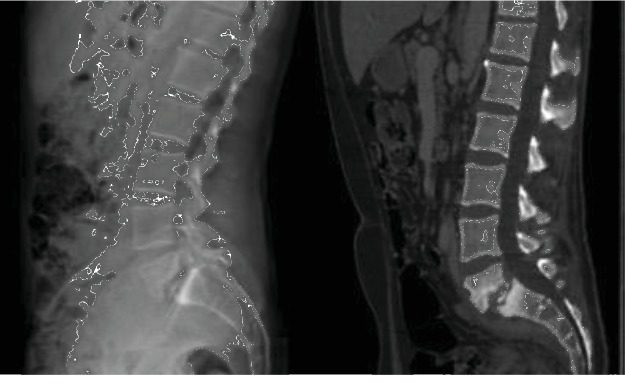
Preoperative (a) lumbar spine radiography in standing position and (b) lumbar CT scan, demonstrating the L5-S1 spondylodiscitis on previous spondylolisthesis, with major osteolysis of the vertebral endplates.
